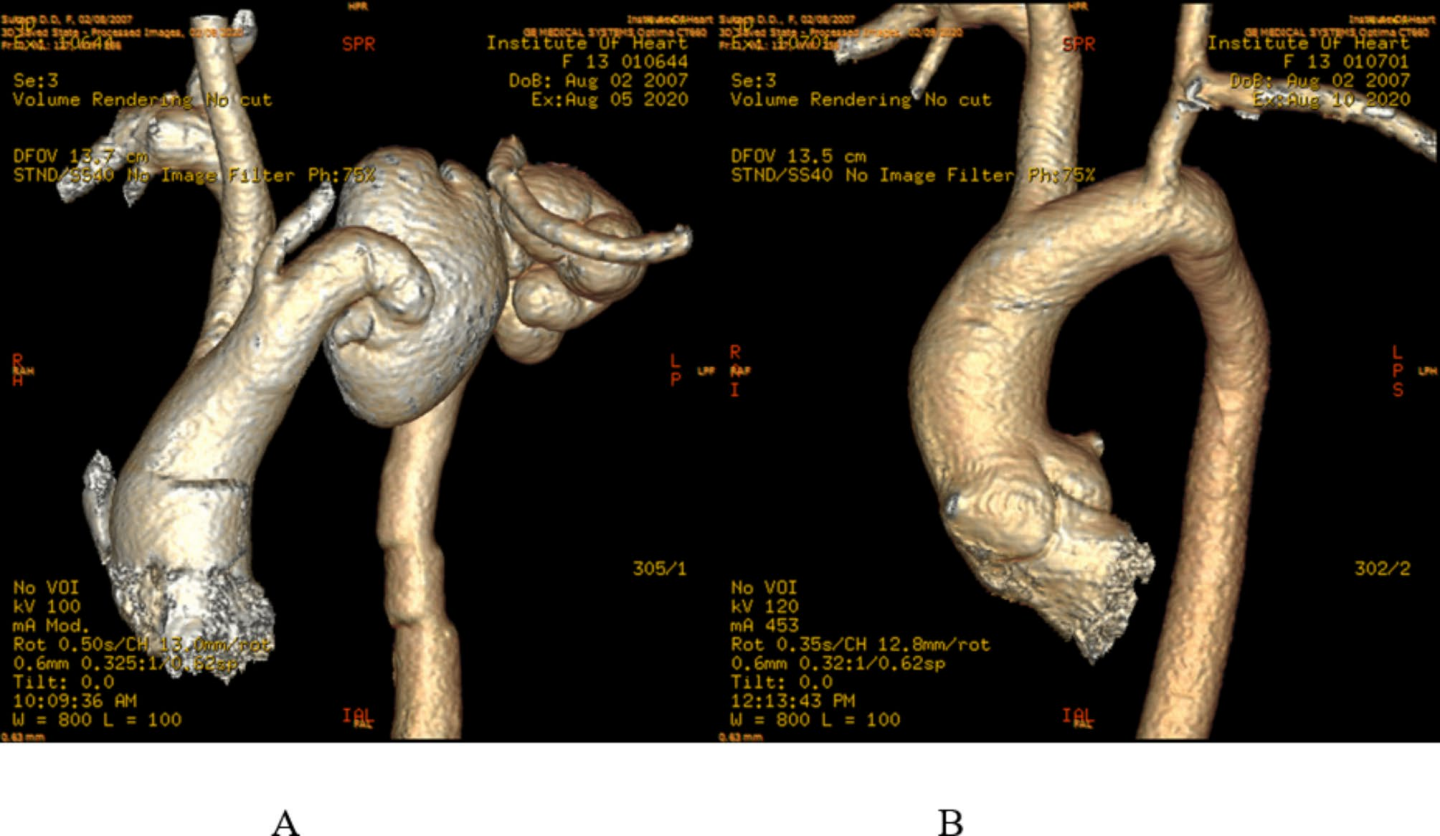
A) 3D reconstructed pre-operative CT angiogram; B) 3D reconstructed post-operative CT angiogram
